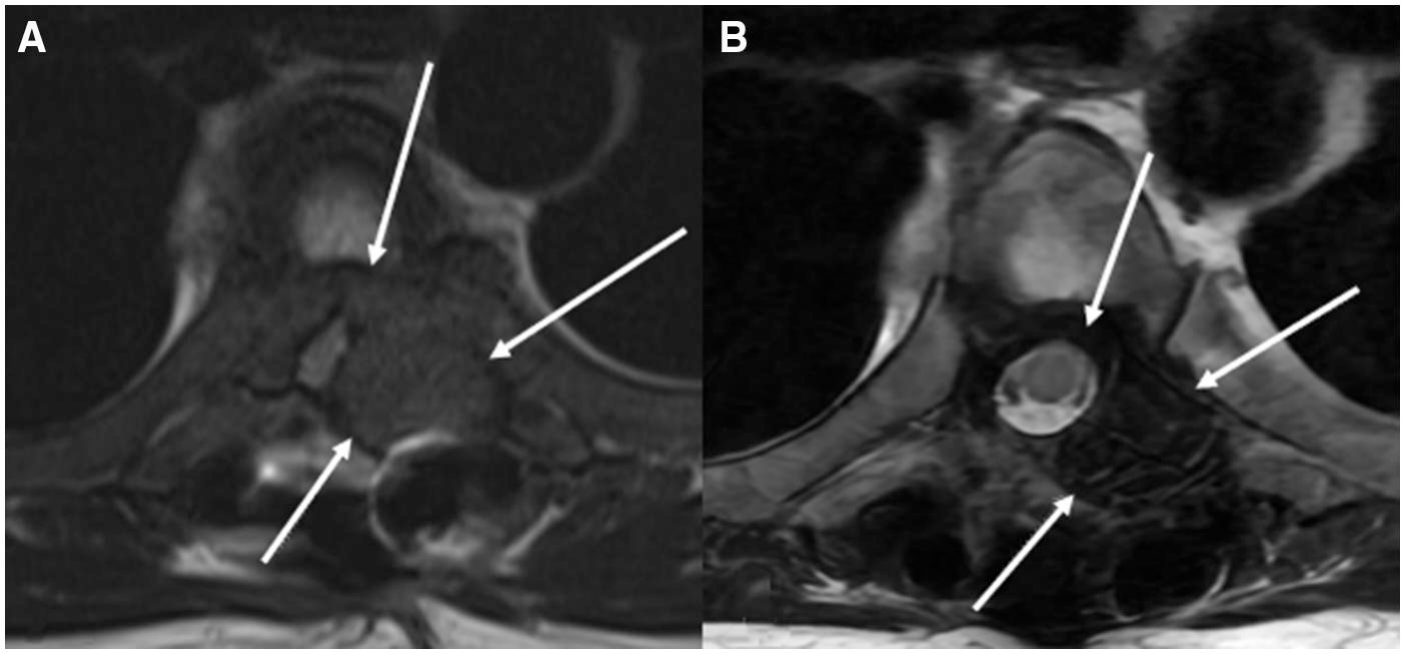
Magnetic resonance imaging (MRI) of the thoracic spine demonstrating prior T4-T6 posterior fusion with T5 laminectomy and T5 tumor debulking with interval worsening of 2.5 × 3.7 cm soft tissue neoplasm resulting in severe canal stenosis and cord compression/edema with near-complete collapse of the T5 vertebral body (A). MRI of the thoracic spine obtained 7 months after parathyroidectomy, demonstrating significant decrease in the size of the soft tissue tumor at T5 without signs of spinal canal stenosis (B).
